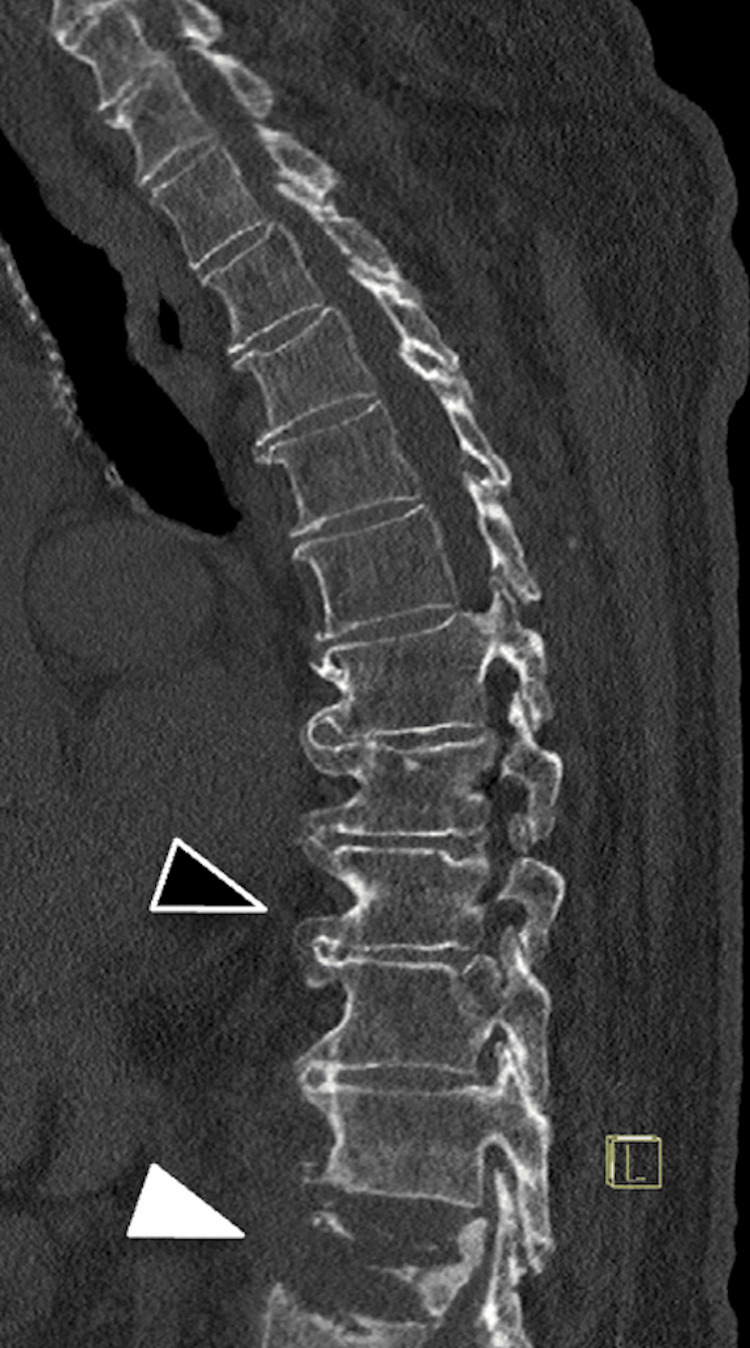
Preoperative CT thoracic spine. Preoperative CT thoracic spine of this 79-year-old man, acquired two days preoperatively, demonstrating a T12 compression fracture (white arrowhead), diffuse idiopathic skeletal hyperostosis (DISH) of the T7-T11 vertebrae, and a T9 vertebral body (black arrowhead) with no evidence of fracture or injury.
